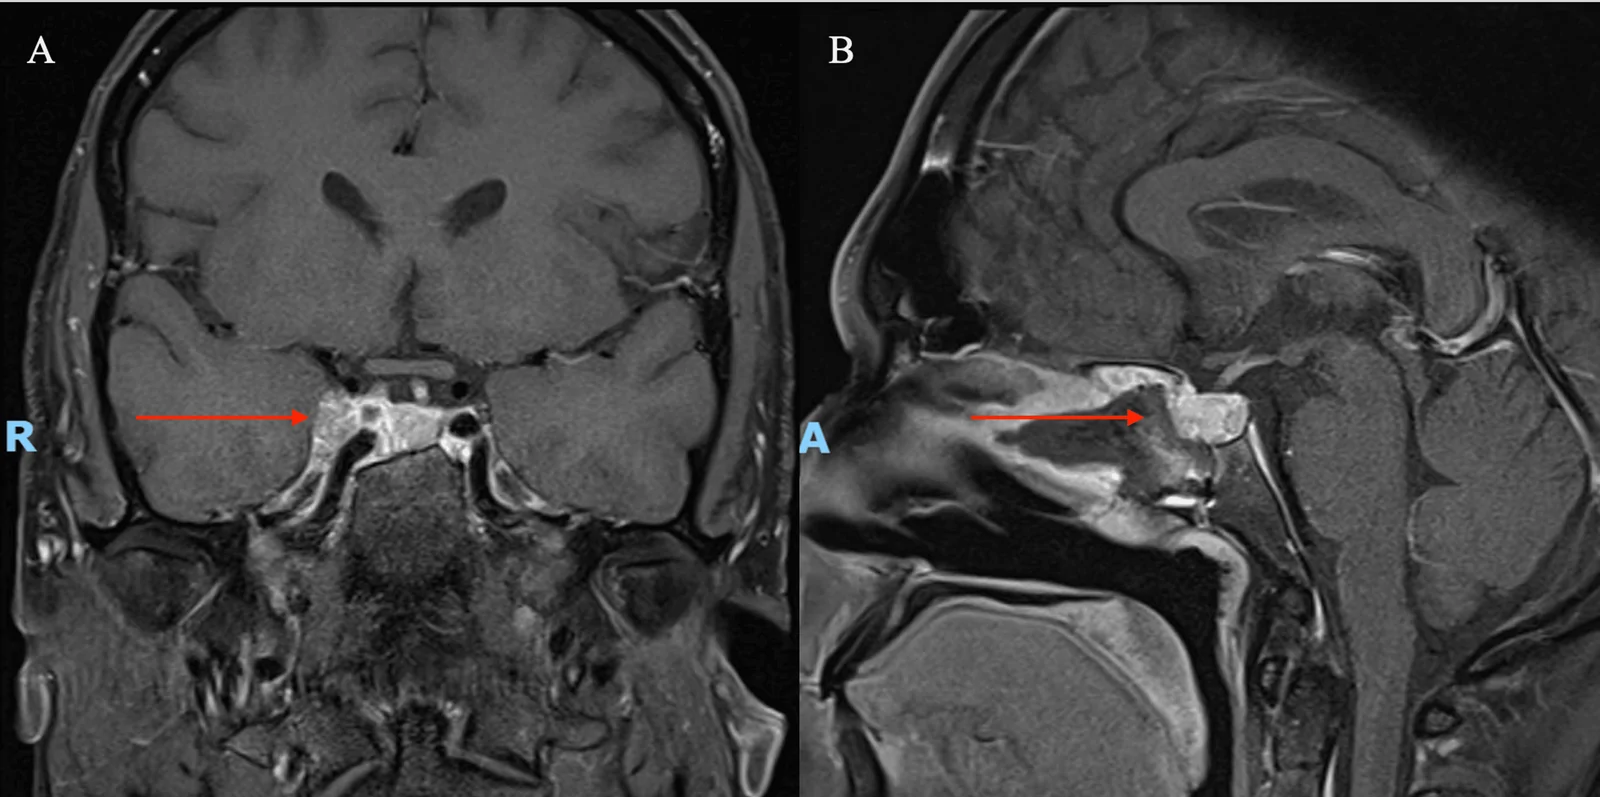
Postoperative images (T1-weighted with contrast coronal (A), sagittal (B)) showing residual tumour lateral to the right internal carotid artery, with reduced suprasellar extension and relieved mass effect on the optic chiasm.
